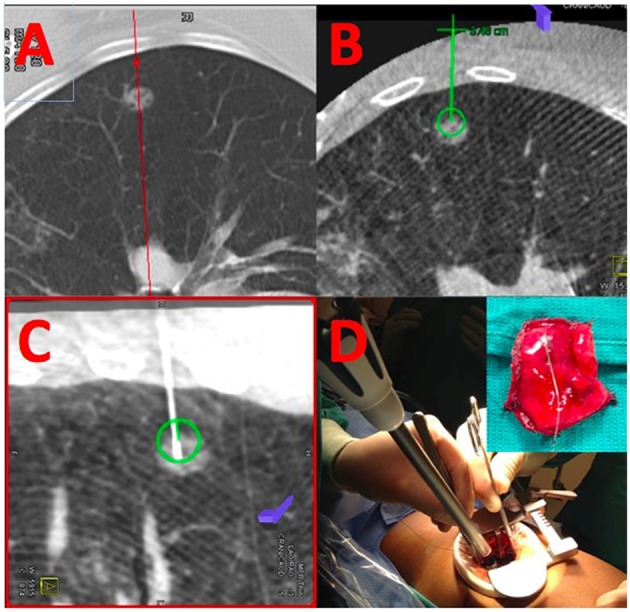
Localization and resection of peripheral GGO. (A) Shows a cross-sectional MDCT image of a peripheral ground-glass opacity. The same nodule is visualized by cone-beam CT (B) and an appropriate needle trajectory is planned (green line). Using laser-beam guidance, the needle is introduced into the GGO (C) using the previously planned trajectory; coils are placed within the lesion, and extending toward the parietal pleura. (D) The lesion is resected using a VATS approach using the coils as a landmark for resection.
